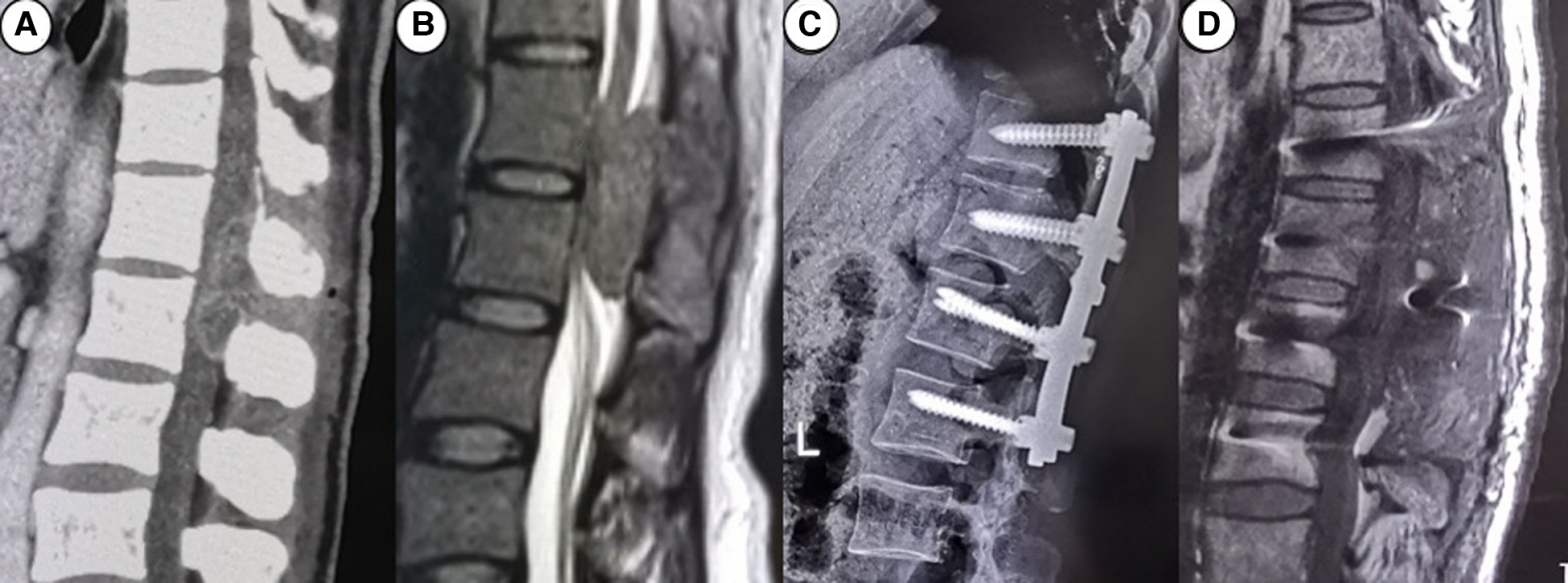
A 51-year-old woman with lung cancer metastases to the T11,12 posterior column, combined with epidural spinal cord compression. (A) the preoperative CT image; (B) the preoperative MRI image; (C) the postoperative CT image; (D) the postoperative MRI image.
